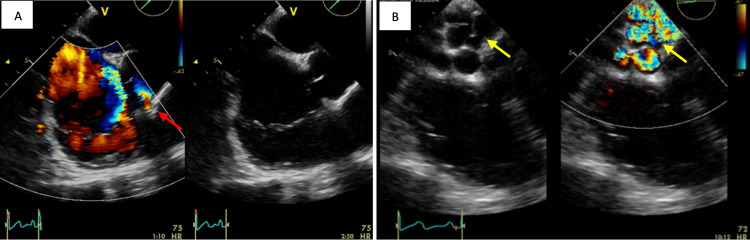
A: Midoesophageal view at 45° showing color Doppler flow originating from the right coronary cusp (red arrow). B: Midoesophageal view at 0° showing several hollow rounded formations filling the right atrium (yellow arrows)
